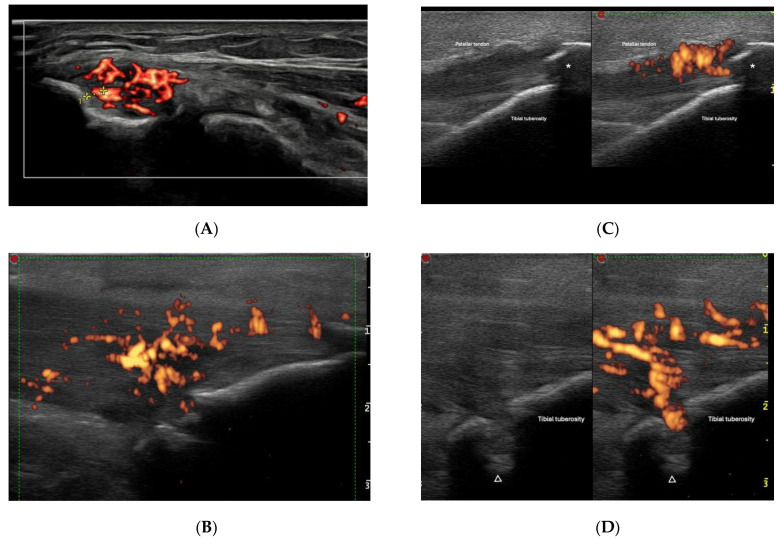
(A) shows the lateral epicondyle longitudinal aspect, with the power Doppler signal in the common extensor tendon (within 2 mm of the cortical bone). The longitudinal aspect of patellar tendon in its tibial insertion is shown in (B–D). Tendon thickening, hypoechoic appearance, and the presence of power Doppler can be observed. Damage aspects, such as enthesophytes (marked with *) and bone erosion (marked with Δ) can be seen [(D) shows the same enthesis twice, in grayscale (left) and with power Doppler (right)].
